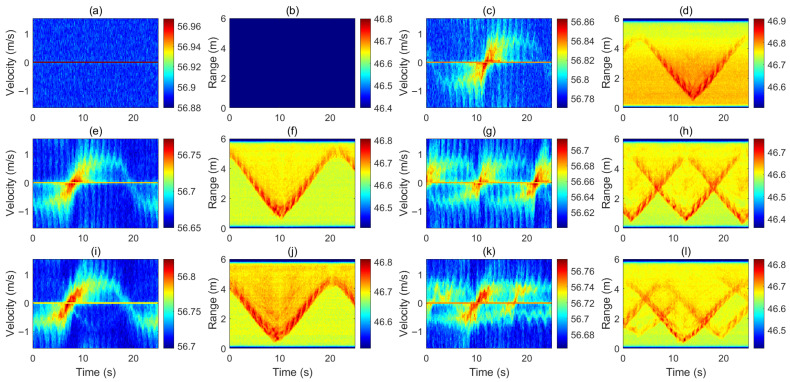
Doppler and range spectrogram measured from each motion state: (a) no-person Doppler, (b) no-person range, (c) one person walking Doppler, (d) one person walking range, (e) two persons (same direction) Doppler, (f) two persons (same direction) range, (g) two persons (toward each other) Doppler, (h) two persons (toward each other) range, (i) three persons (same direction) Doppler, (j) three persons (same direction) range, (k) three persons (random walking) Doppler, (l) three persons (random walking) range.
